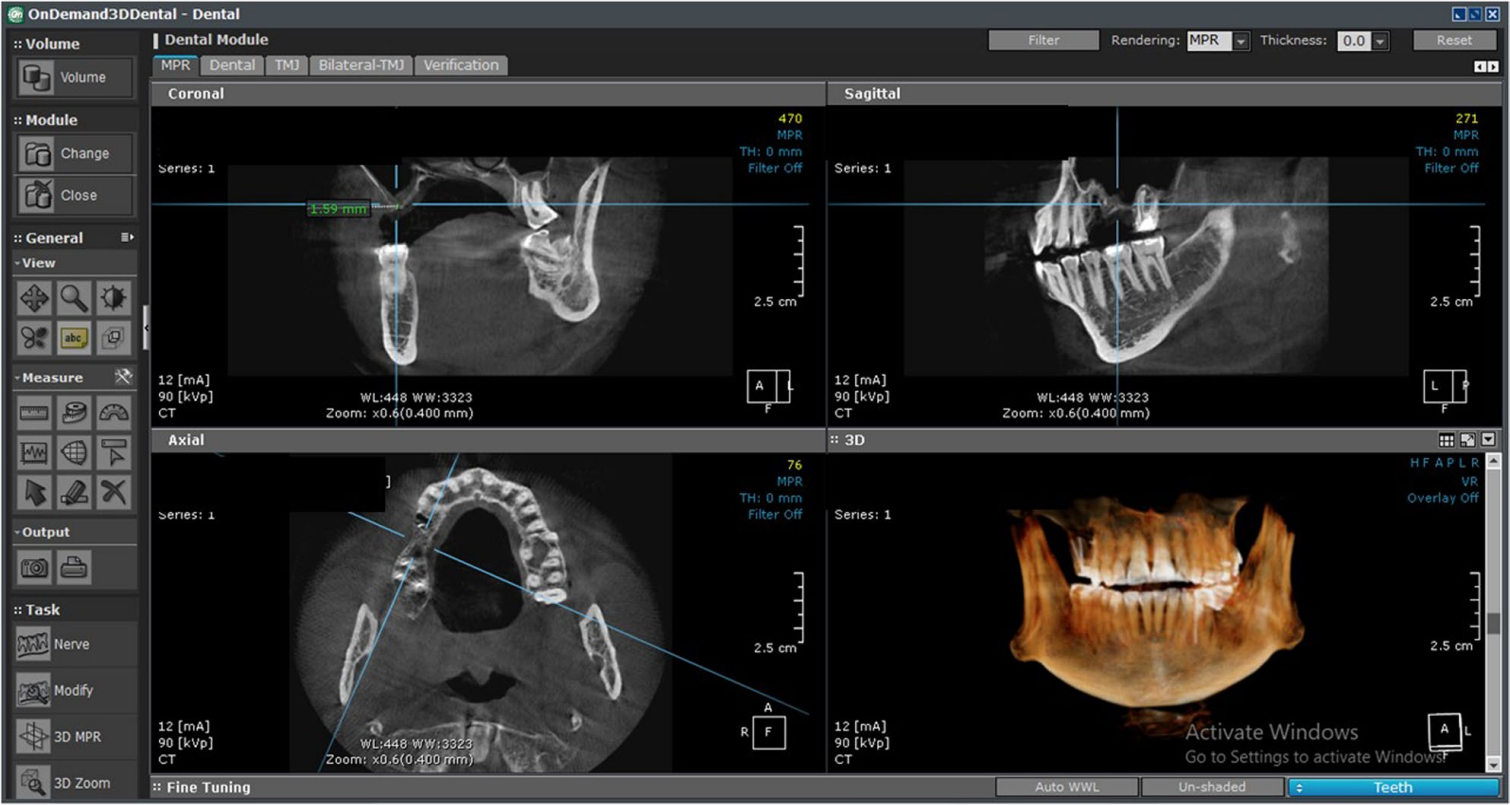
Preoperative CBCT showing the residual bone height in the left maxillary posterior partially edentulous area
